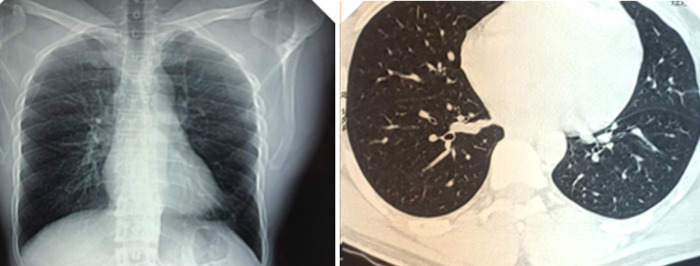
chest radiograph and CT scan at the end of therapy shows no abnormality
